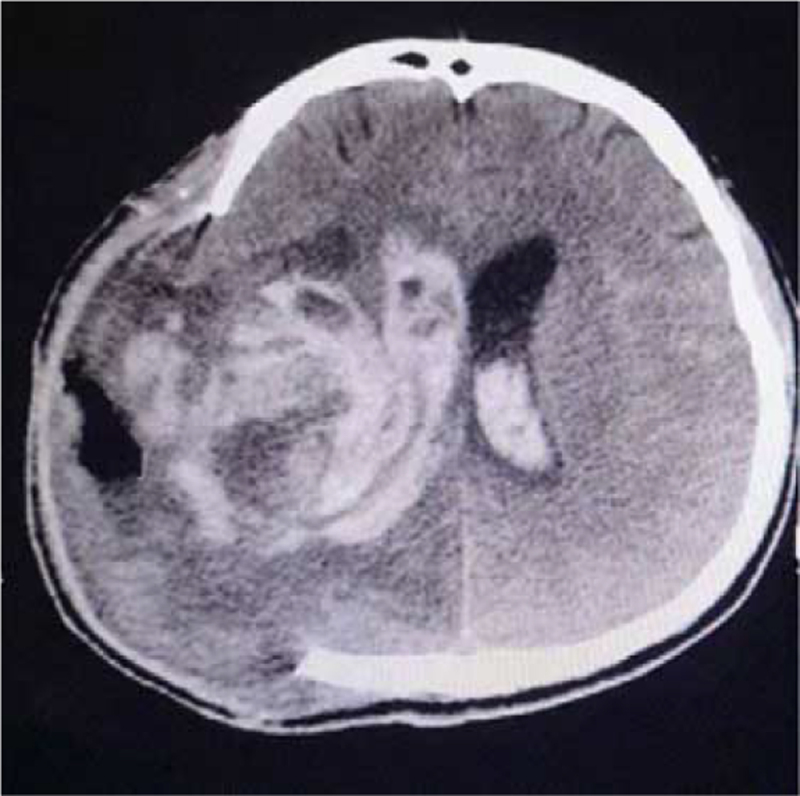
Patient's imaging data during the fifth ICH. The head CT showed right ICH in the same position, which penetrated the bilateral ventricular system. CT = computer tomography, ICH = intracerebral hemorrhage.
